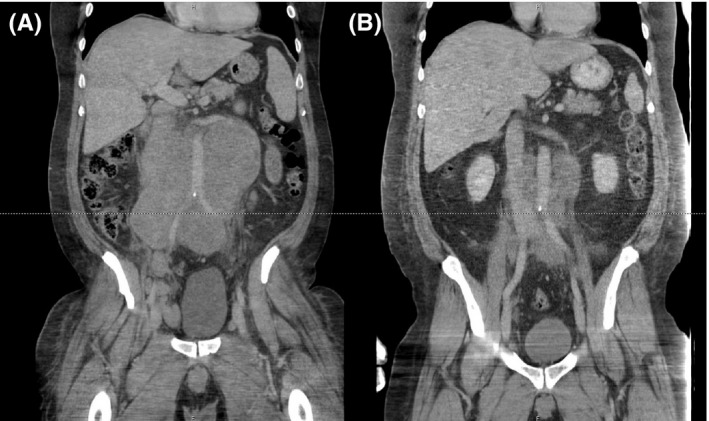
CT scans of the neck, chest, abdomen, and pelvis demonstrated marked retroperitoneal lymphadenopathy (A); this presented as testicular swelling. There is significant improvement after ten weeks (three cycles) of R‐CHOP chemotherapy (B)
